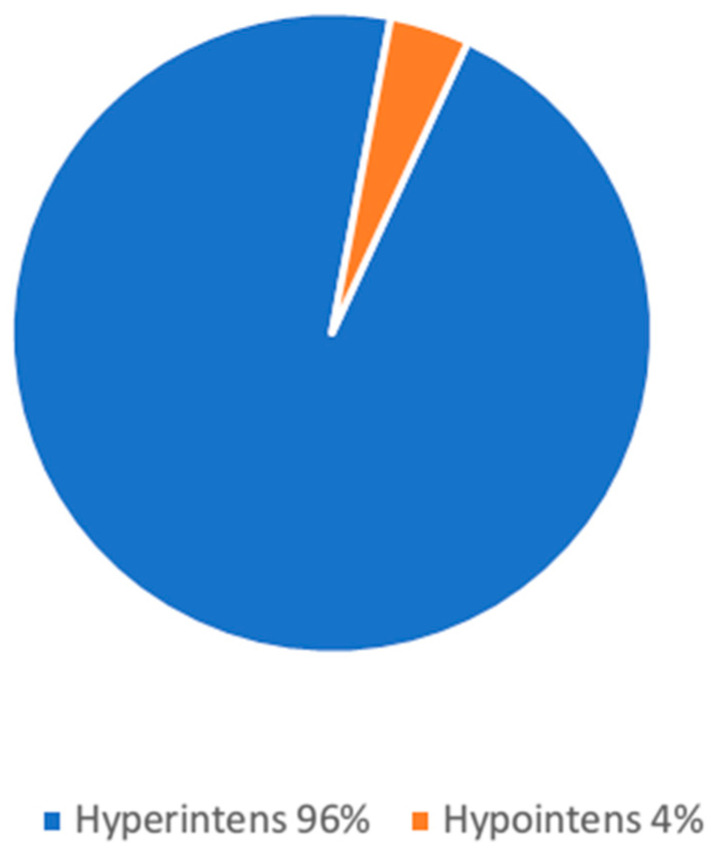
Distribution of liver lesion intensity on T2-weighted images.
